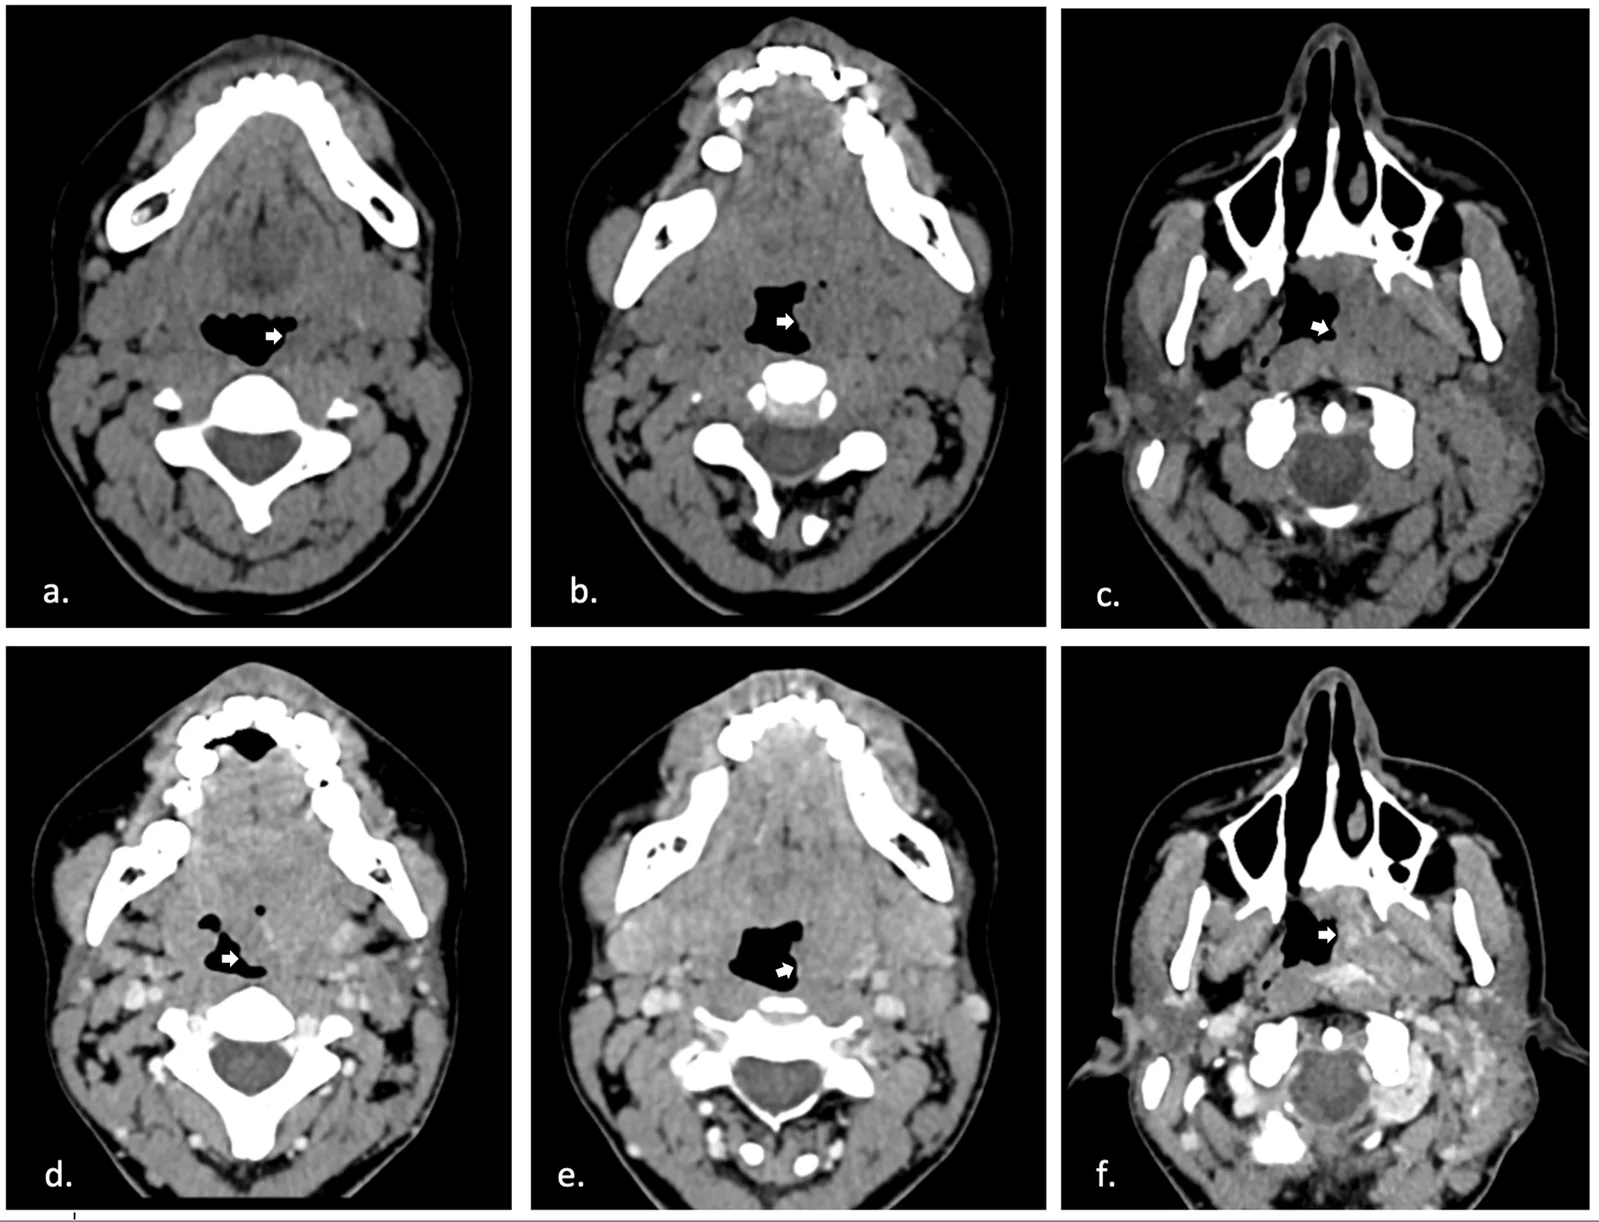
Contrast-enhanced CT Axial non-contrast (a–c) and contrast-enhanced (d–f) computed tomography of the paranasal sinuses and maxillofacial region demonstrates a heterogeneous soft-tissue mass centered within the left pharyngeal mucosal space, extending across the nasopharynx and oropharynx. Following intravenous contrast administration, the lesion exhibits heterogeneous enhancement, consistent with a vascular neoplasm.
